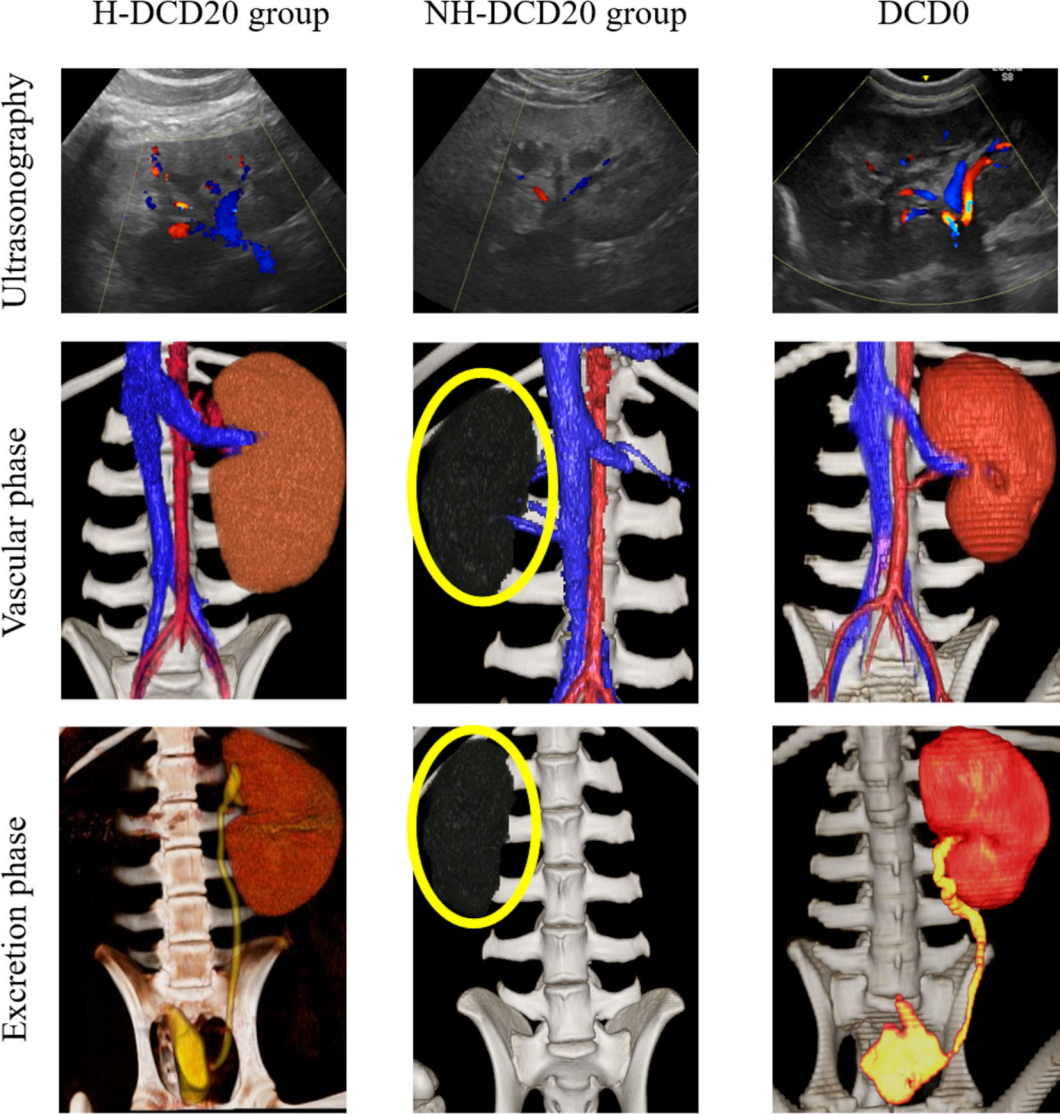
Ultrasonographic and contrast CT images. Upper row: Ultrasound images on the first day after the operation are shown. Renal blood flow in H-DCD20 individuals is clearly confirmed; however, some areas are difficult to see with a Doppler. Almost no renal blood flow is observed in NH-DCD20 individuals, and the renal parenchyma shows a hypoechoic image similar to that of surrounding tissues. Kidney blood flow in DCD0 is clearly confirmed, and branching of interlobar arteries and veins is observed from segmental arteries and veins. Middle/lower row: contrast-enhanced CT is used to demonstrate the vascular and excretory phases, and 3D stereoscopic images are shown. In H-DCD20 and DCD0, inflow of contrast medium into the kidney is observed, and the ureter and bladder are imaged. However, in individuals of NH-DCD20 and H-DCD20 without blood flow, the renal arteries are not imaged, and the ureter and bladder are not imaged during the excretory phase (yellow circle, transplanted kidney). CT, computed tomography; 3D, three-dimensional. *H-DCD20, n = 4; NH-DCD20, n = 3; DCD0, n = 1.
